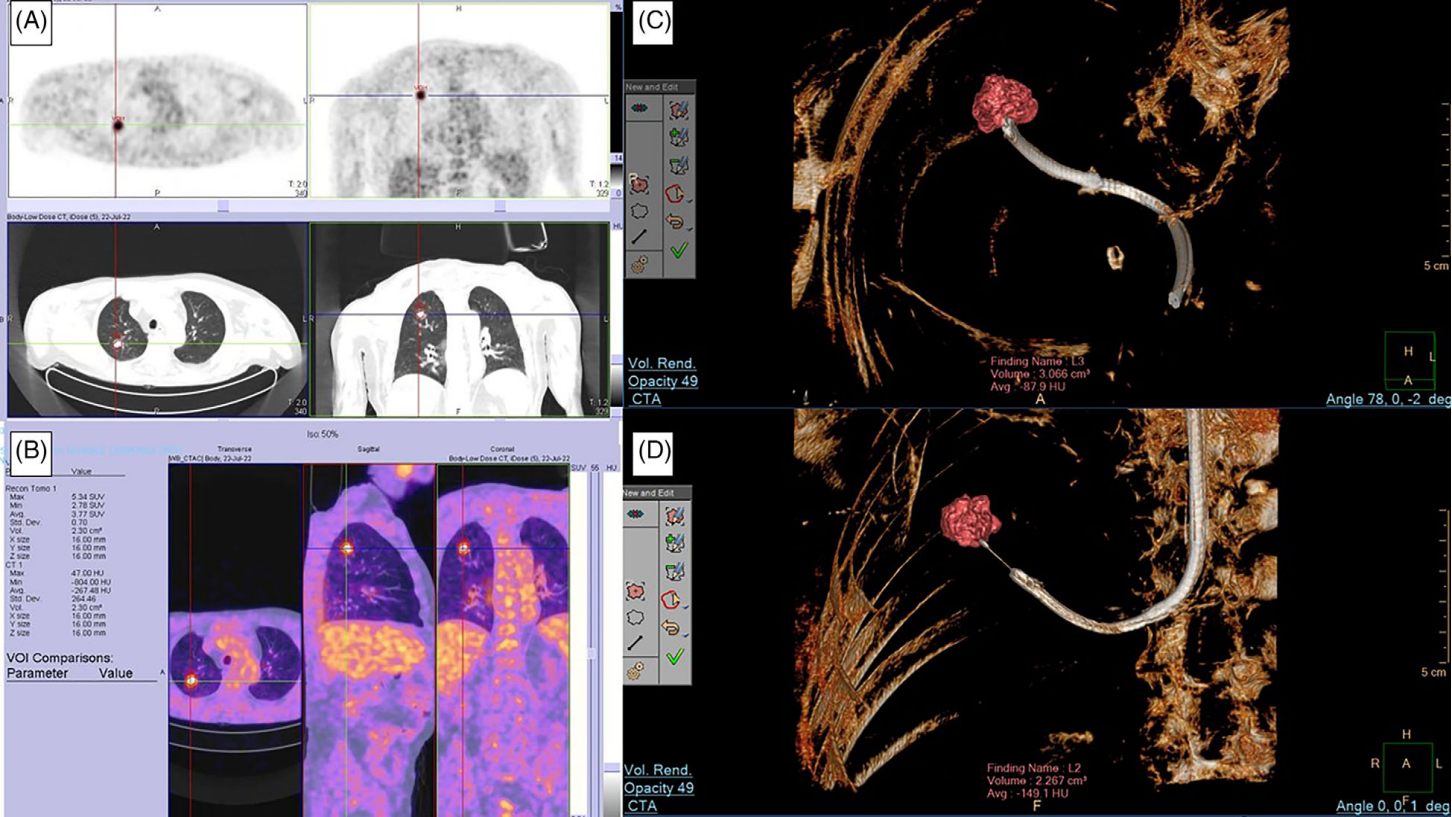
(A and B) 18F‐fluorodeoxyglucose (FDG) PET/CT uptake in correspondence of the right upper lobe; (B and C) aScope 5 Broncho HD 5.6/2.8 single‐use bronchoscope reach the lesion guided by 3D fluoroscopy CIOS guidance system: TBNA (C) and forceps (D) sampling (B).
